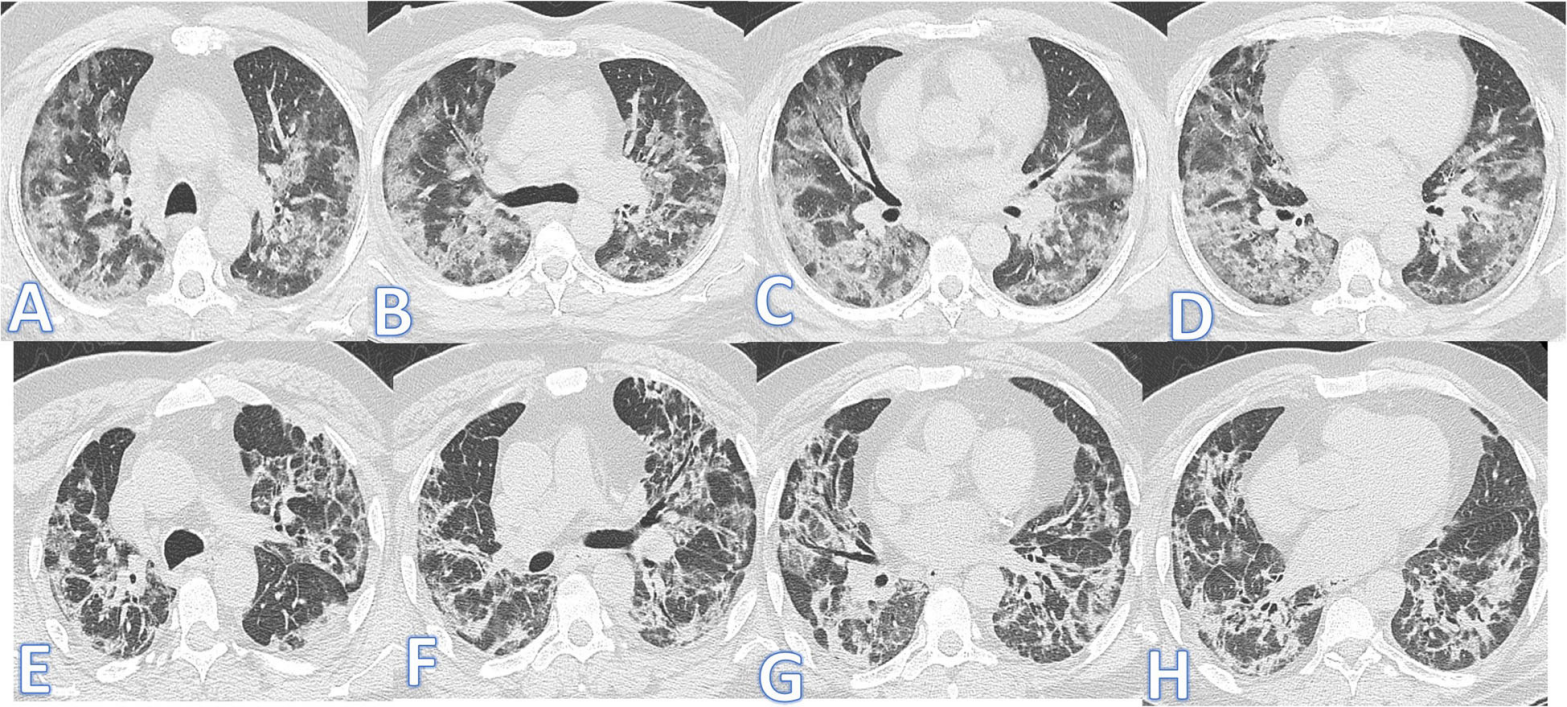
Fifty-five-year-old male: he has DM, HTN, and IHD; peak CT severity score 16; consolidation/crazy-paving score 14. He was admitted to the ICU; laboratory results showed lymphopenia, high CRP, d-dimer, normal serum ferritin, and sensitivity troponin. Steroid was given; length of stay during hospitalization is 30days. Peak CT during admission (a–d) showed bilateral consolidation patches more peripheral and at the lower lobes with a crazy-paving appearance. Follow-up CT 3weeks after discharge (e–h) showed lung fibrosis with parenchymal bands, coarse reticular pattern, irregular interface, and mild traction bronchiectasis at the anterior segments of both upper lobes and the medial segment of the right middle lobe
